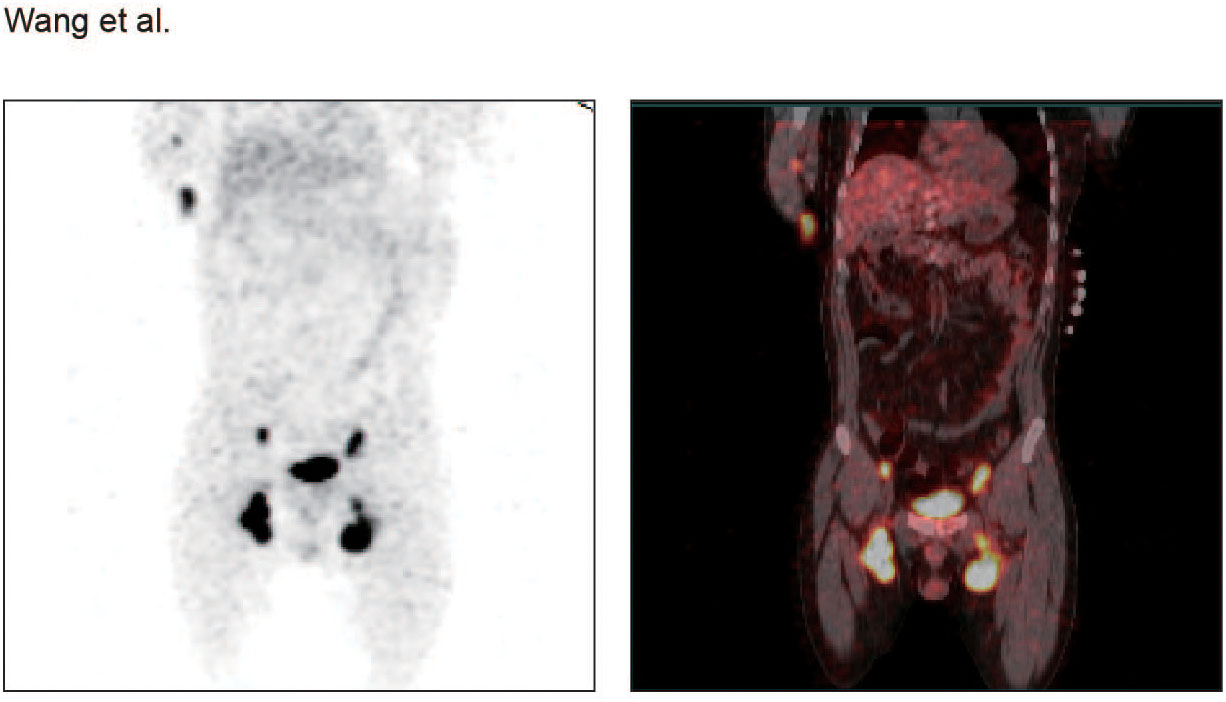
PET-CT imaging of the patient.
